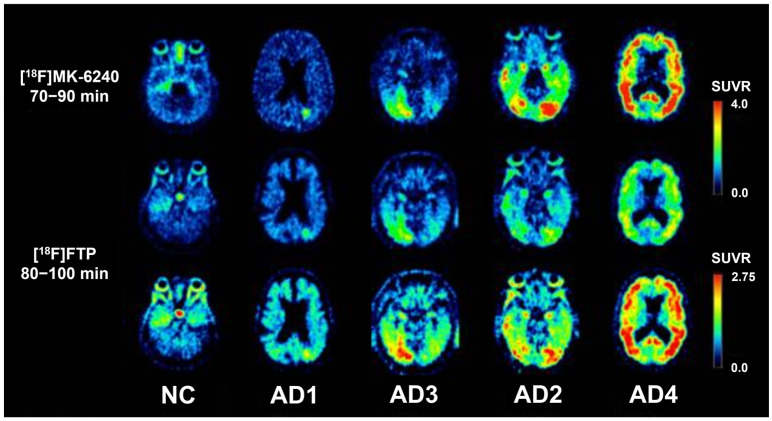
Tau tracers ([^18^F]MK-6240 and [^18^F]FTP), scaled by tracer-specific standardized uptake value ratios (SUVRs), visibly differentiate individuals with low tau and normal cognition (NC) from those with high tau in AD (AD1, AD3, AD2, AD4). This research was originally published in JNM. Alexandra Gogola, Davneet S. Minhas, Victor L. Villemagne, Ann D. Cohen, James M. Mountz, Tharick A. Pascoal, Charles M. Laymon, N. Scott Mason, Milos D. Ikonomovic, Chester A. Mathis, Beth E. Snitz, Oscar L. Lopez, William E. Klunk, and Brian J. Lopresti. Direct Comparison of the Tau PET Tracers 18F-Flortaucipir and 18F-MK-6240 in Human Subjects. J Nucl Med. Jan 2022, 63 (1) 108-116. © SNMMI.
